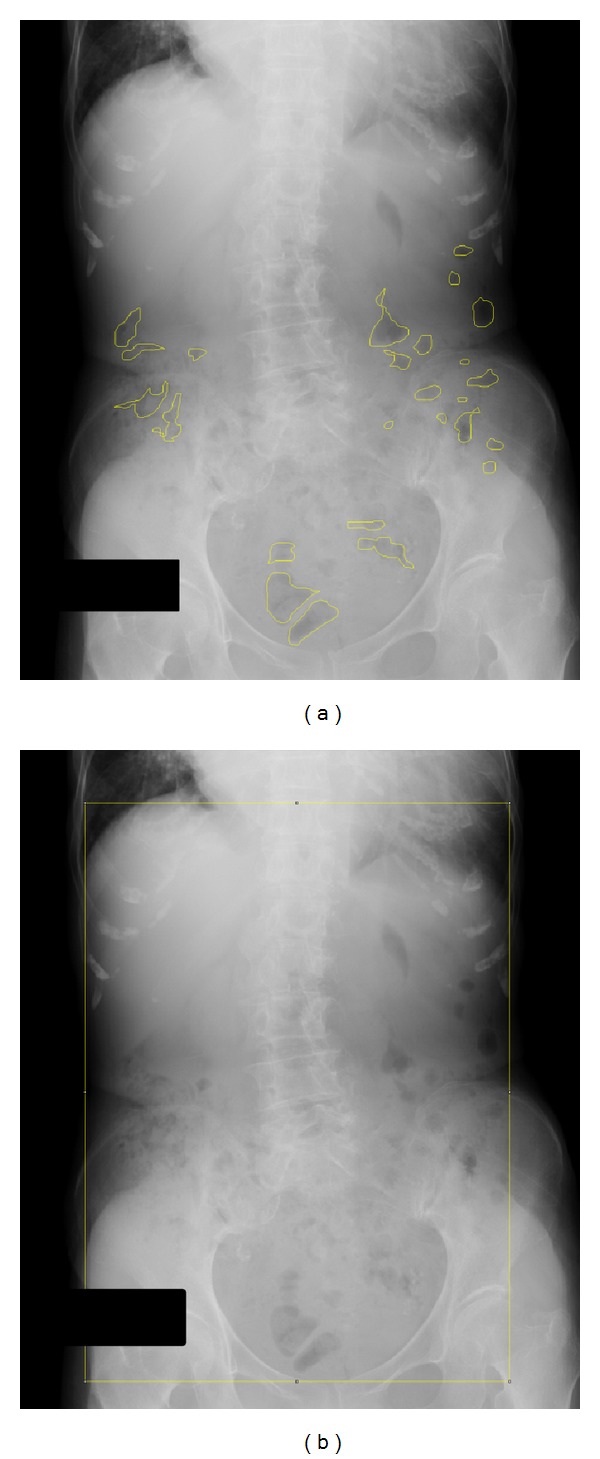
Estimation of gas volume score (GVS). Plain abdominal radiographs obtained from fasting subjects were converted to digital data. The data were read using ImageJ, an image analysis program, and intestinal gas was traced using the program. (a) Tracing image and pixel count of the gas was 3,533 in this patient. (b) The window of abdominal area. The rectangular area was measured as the area between the inferior right side margin of the diaphragm, the inner costal margin, and the superior border of the pubic symphysis. The pixel count of the rectangular area was calculated as 92,968 in (b). GVS was calculated as (a)/(b)%; therefore, the GVS of this image is “3,533/92,968 = 0.038(3.8%).”
